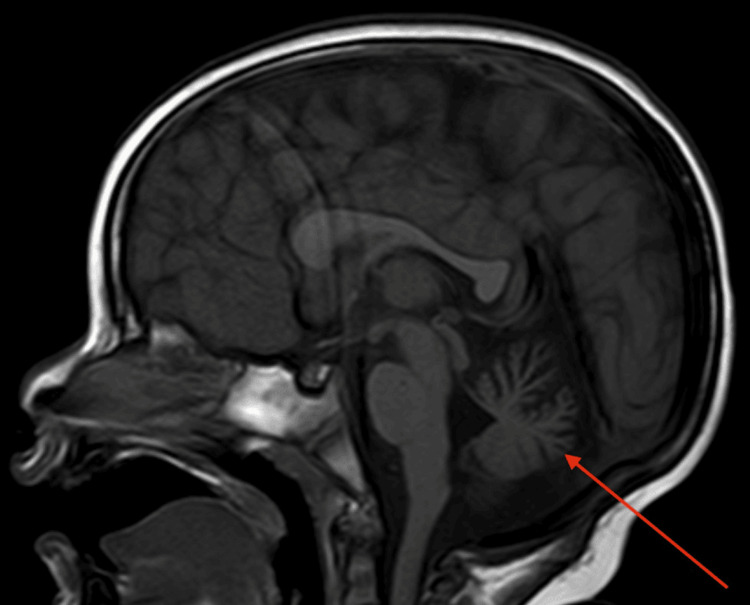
Brain MRI scan of the patient. Red arrow: the cerebellum showing vermis hypoplasia.
